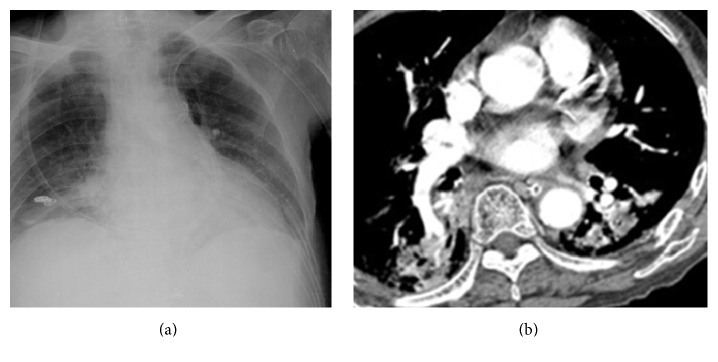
Mixed central and peripheral thrombotic and parenchymal pulmonary lesions. The patient is an 87-year-old women with hepatocellular carcinoma. She felt dyspnea. A chest X-ray (a) shows a low right lung lesion. A CT scan (b) reveals abnormal tumor/thrombotic lesions within the right superior pulmonary vein with extension into the left atrium and into the peripheral lung parenchymal tissue.
